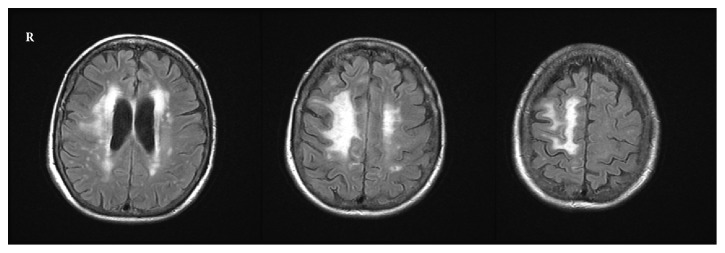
MRI with contrast shows multifocal T2 hyperintense abnormalities with the largest focus in the right frontal lobe.
